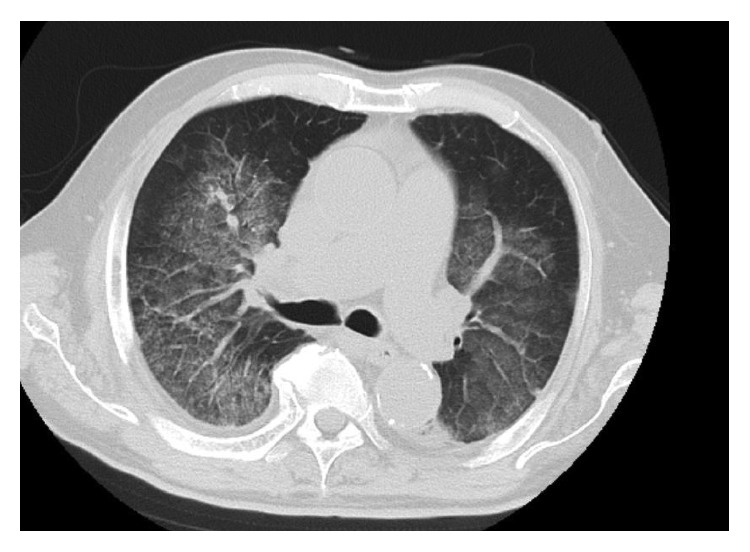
Chest computed tomography findings on admission. High-resolution computed tomography of the chest shows reticular opacity with bronchial-wall thickening and interlobular septal thickening in both lung fields.
